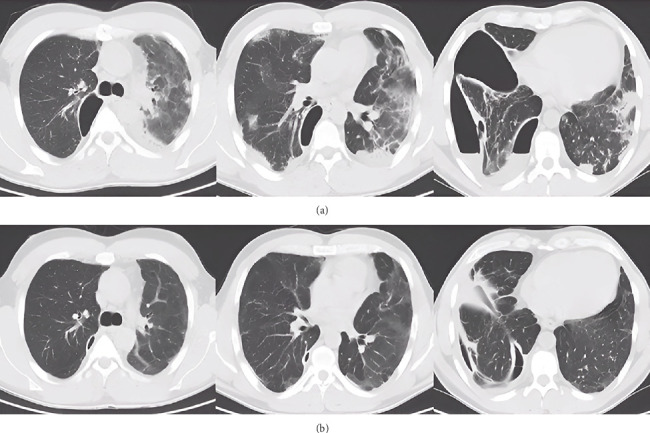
Follow-up CT scans of the patient's chest (Case 1) performed on the (a) 20th of February 2024 and (b) 27th of March 2024.
